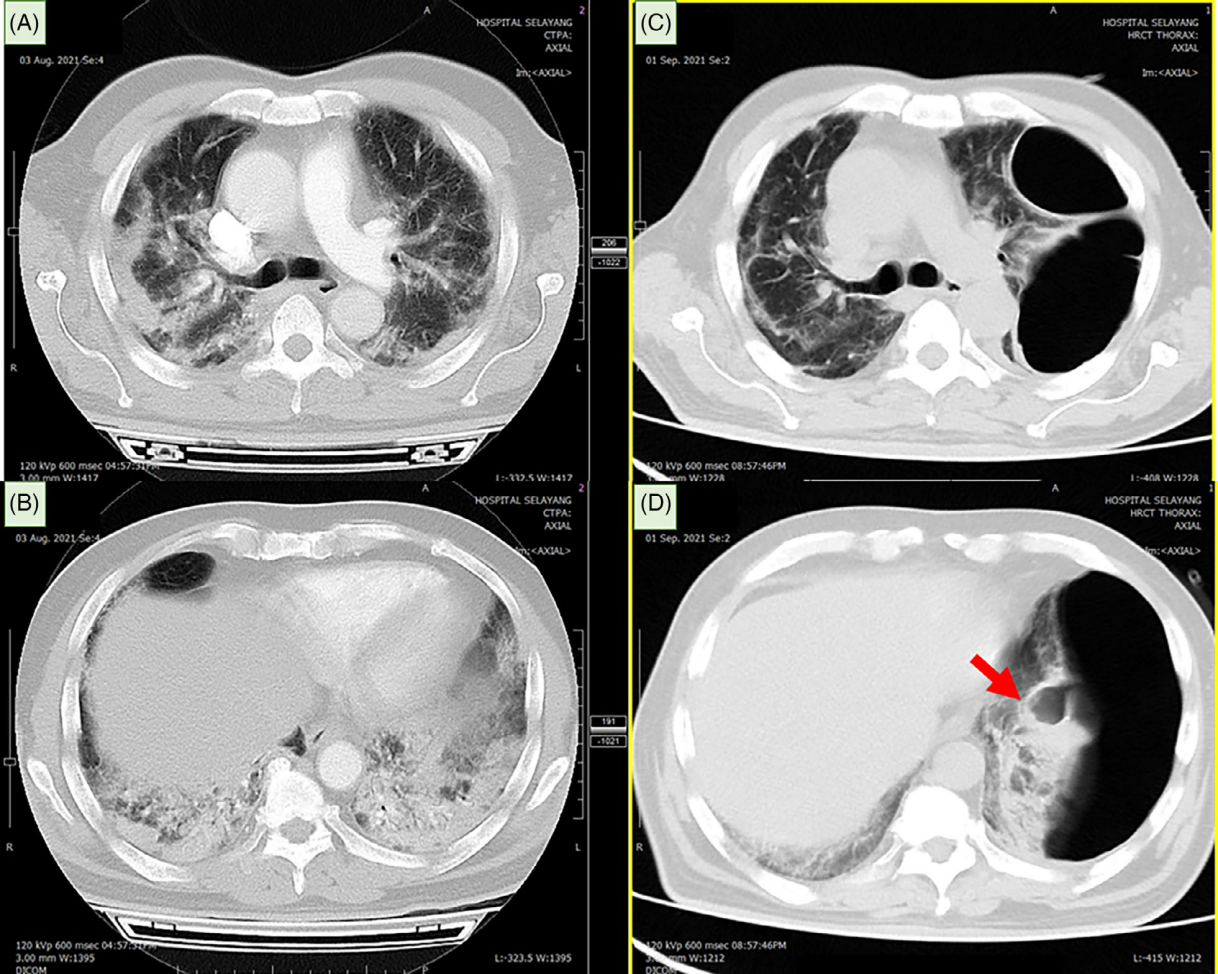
CT thorax at day 4 of SARS‐CoV‐2 infection (image A and B) revealed patchy consolidation in both lungs, predominantly at bilateral peripheral and lower lobe distribution. A repeat CT thorax at 1 month post SARS‐CoV‐2 infection (image C and D) showed bullous development with ruptured left lower lobe bullae leading to loculated pneumothorax (red arrow). The compressive effect of pneumothorax contributed to left lower lobe collapse and mediastinal shift to the right.
